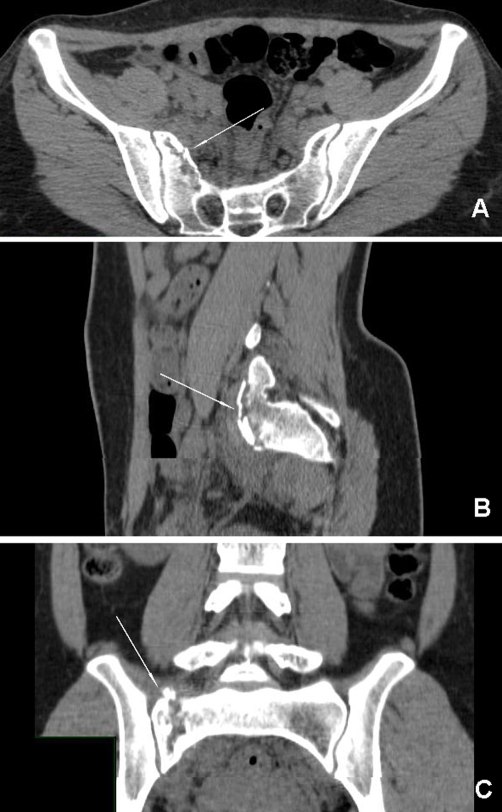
Axial (A), sagittal (B) and coronal (C) reformatted CT images showing incomplete, fracture located on the superior and anterior part of the first sacral vertebra and no disruption of the right sacroiliac joint and the first foramina.
